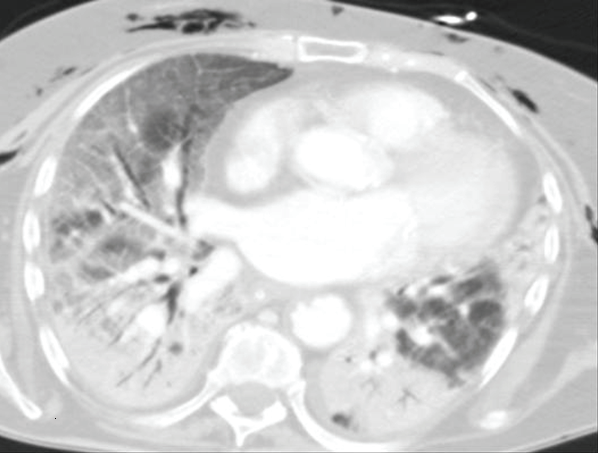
Axial CT chest scan.
